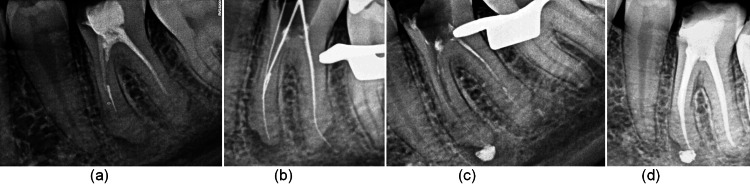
SI removal: (a) intraoral periapical showing previously treated with a separated instrument in mesiobuccal canal of mandibular first molar. (b) SI bypassed with size 8 K-file. (c) Instrument fragment retrieved and calcium hydroxide intracanal medicament removed after two weeks. (d) Intraoral periapical showing obturated mandibular molar.
